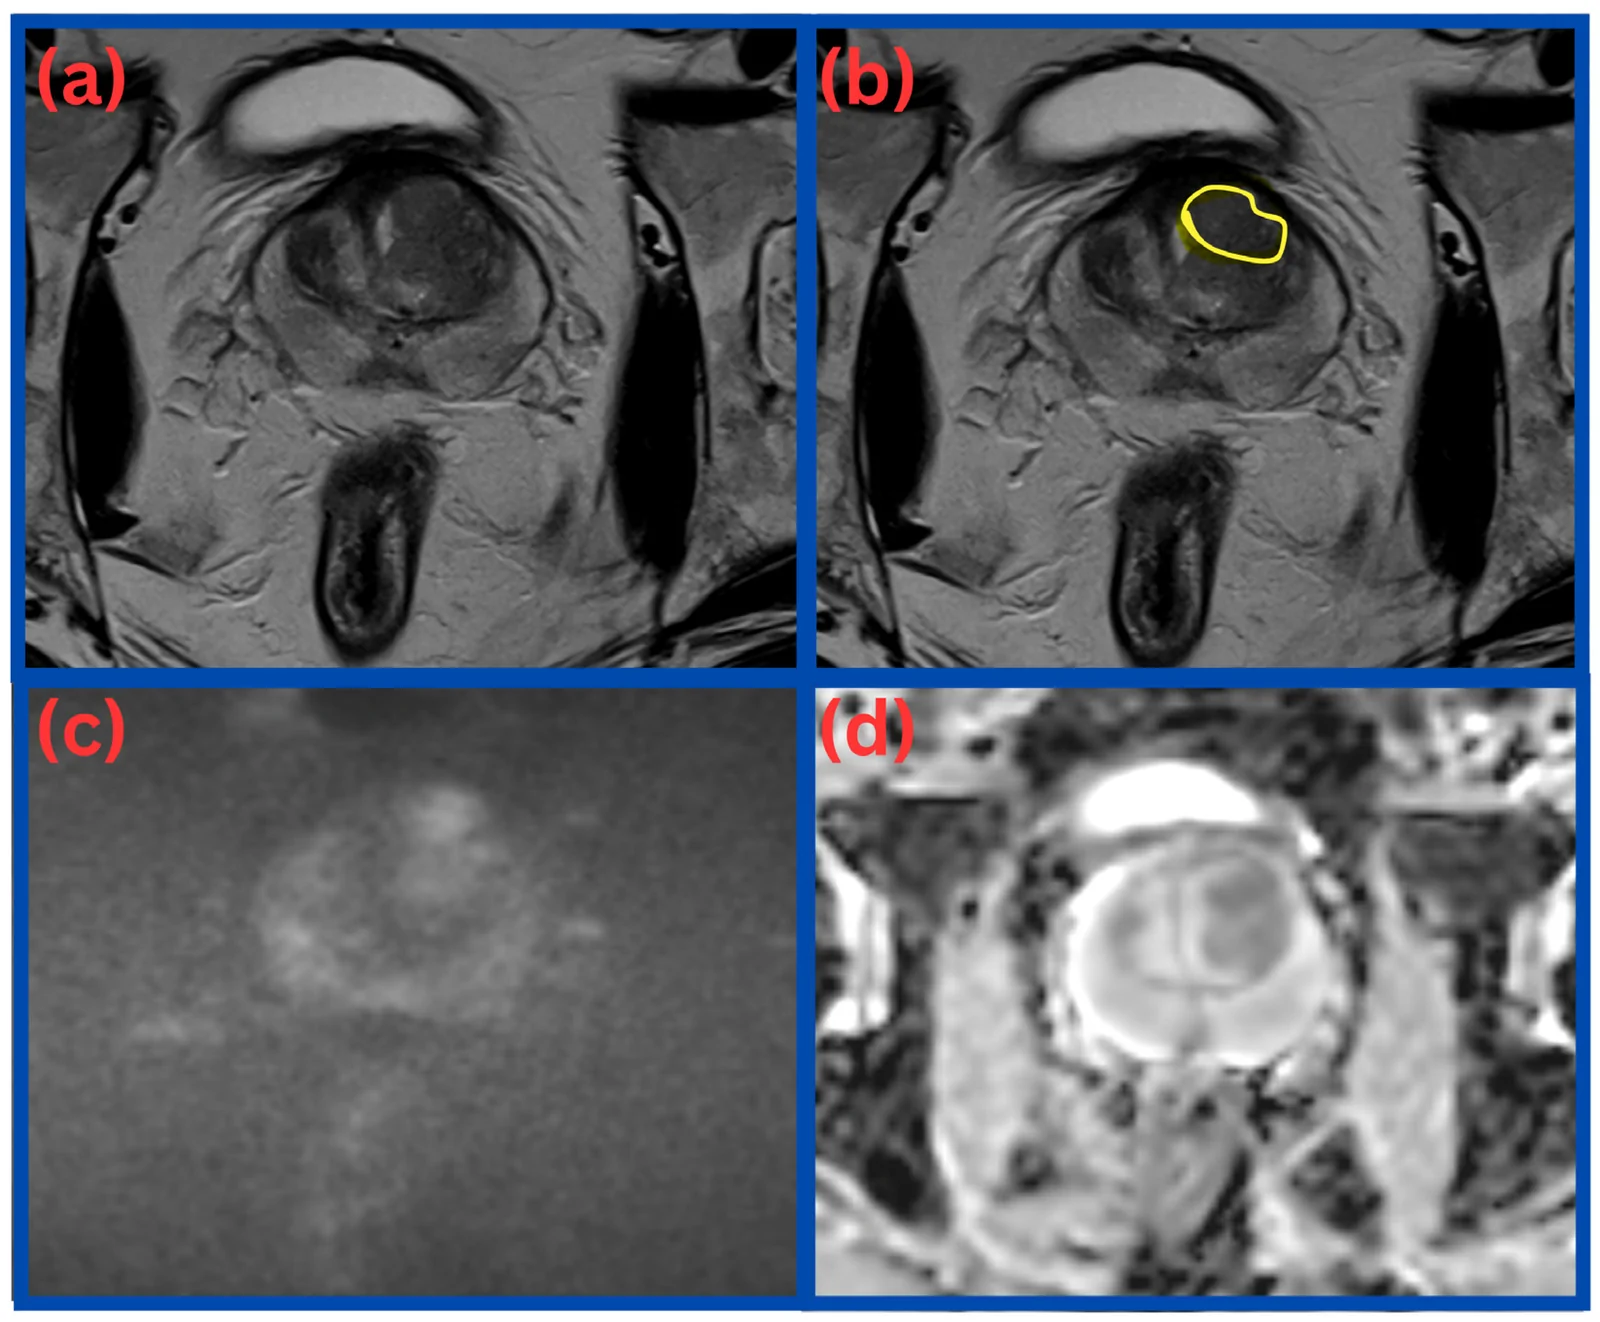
Multiparametric MRI of a PI-RADS 4 lesion. (a) Axial T2-weighted image showing a focal area of T2 hypointensity, and (b) the corresponding axial T2-weighted image with the lesion outlined in yellow. (c) High *b*-value diffusion-weighted image (*b* = 1400 s/mm^2^) demonstrates focal high signal, and (d) the ADC map shows corresponding marked hypointensity, together indicating definite diffusion restriction. The combination of a focal, non-circumscribed lesion with clear diffusion restriction corresponds to a PI-RADS 4 (clinically significant cancer likely) assessment, warranting targeted biopsy.
